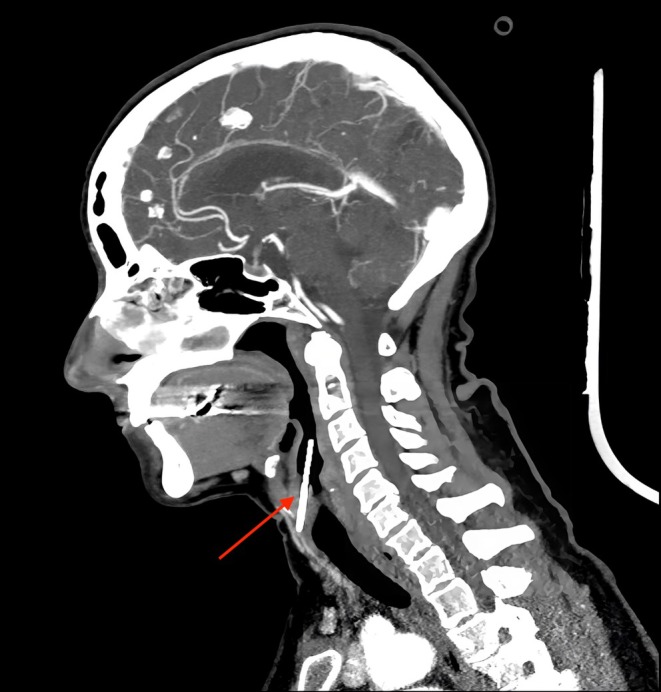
Arterial sagittal computed tomography scan (CT scan) showing the needle penetrating the right vestibular fold, with no evidence of vascular injury.
